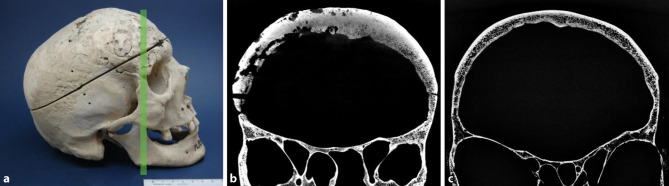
Sclerotic reorganization. Skull of a 43-year-old man presenting with syphilis compared to a 76-year-old male skull without syphilitic signs used as a control. a Photograph of the skull with macroscopically visible signs of syphilitic alterations. The green line indicates the plane of the scan. b Cross-section of the affected part of the skull obtained by µ‑CT showing lytic destructions and a massive sclerotic reorganization compared to the skull of the 76-year-old man in c lacking any infectious lesions used as control
